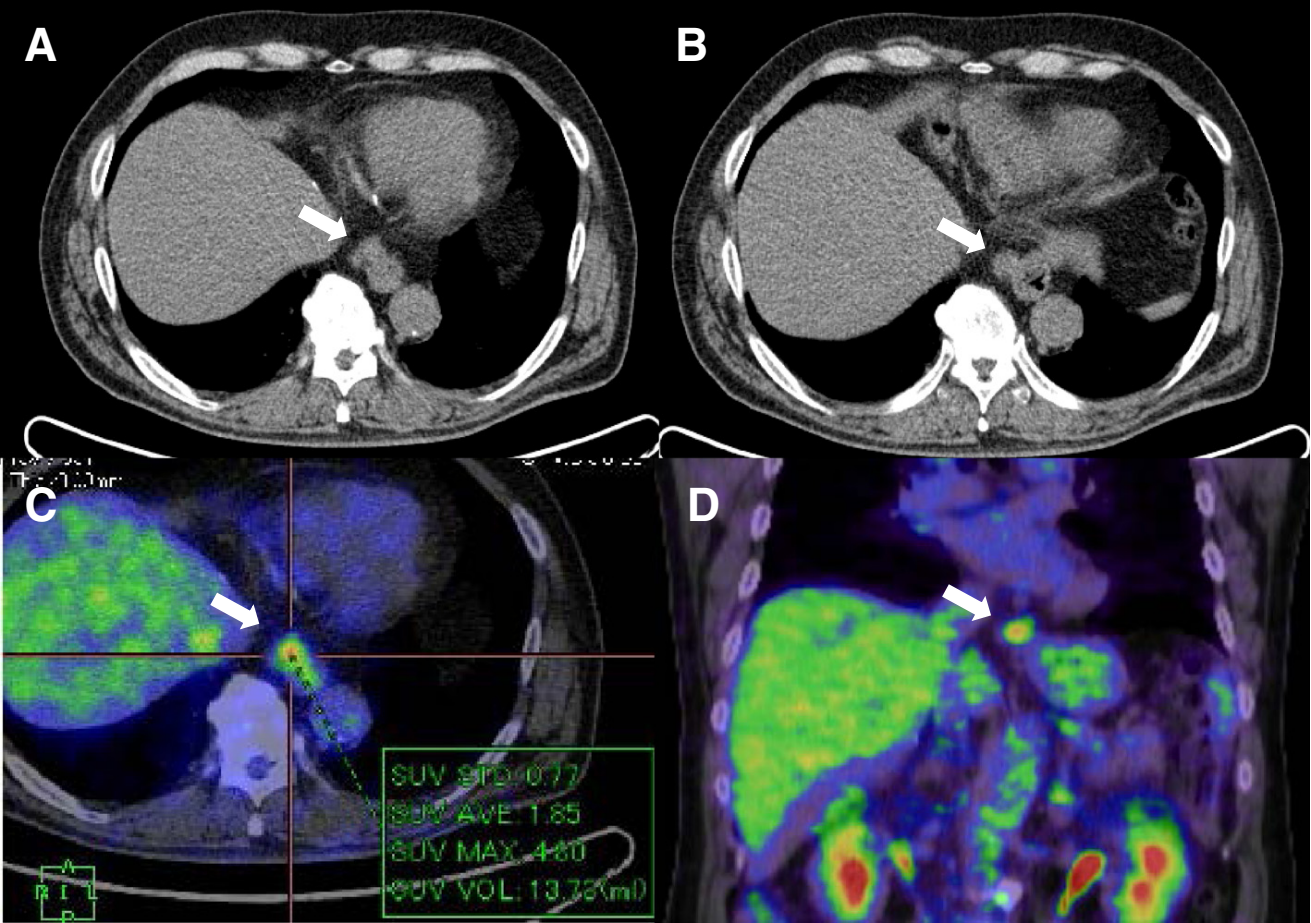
CT and PET-CT findings of posterior mediastinal lymph nodes. (A, B) CT shows multiple enlarged lymph nodes (arrows). (C, D) PET-CT shows significant accumulation (SUVmax, 4.80) in the posterior inferior mediastinum (arrows).
